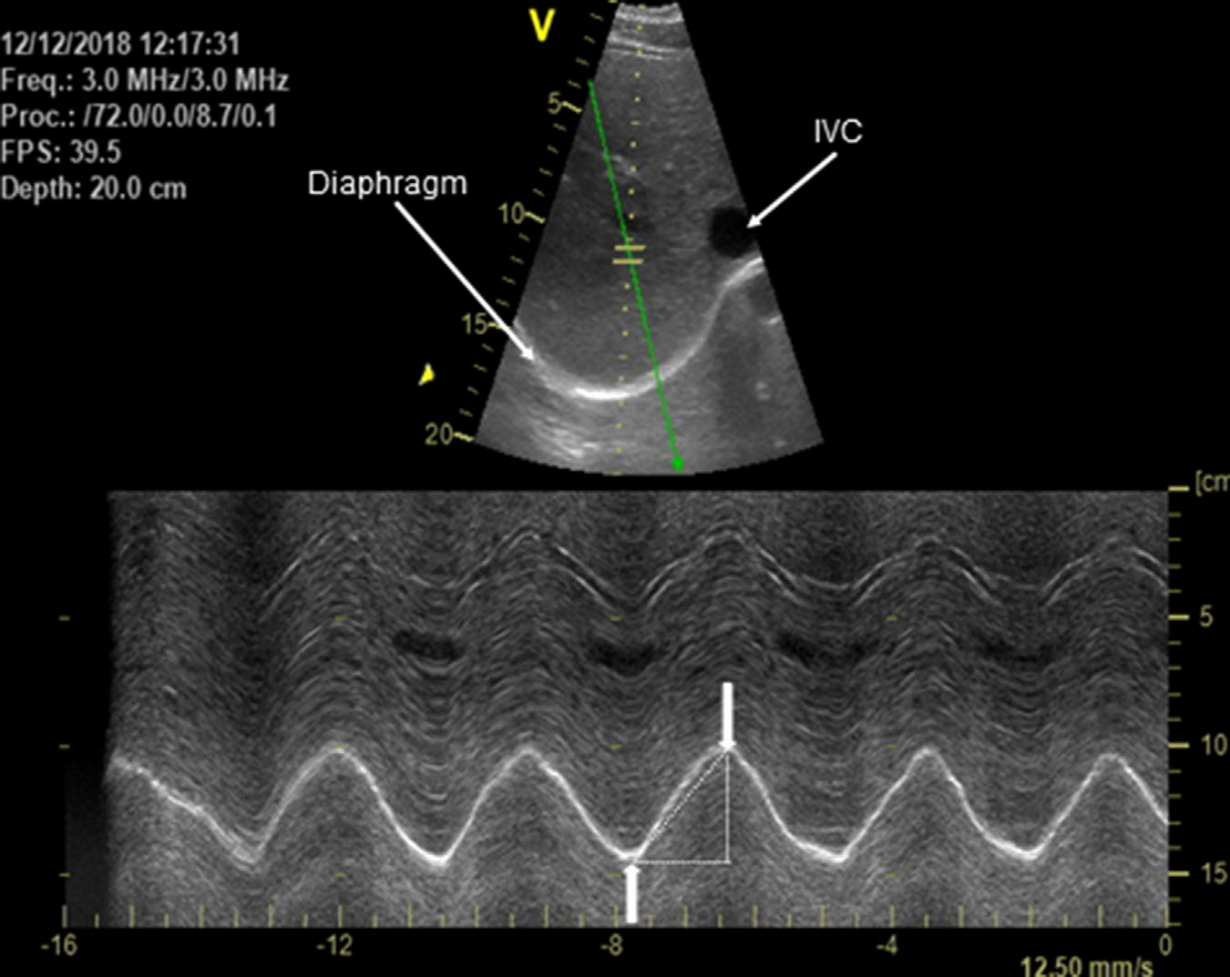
Representative ultrasound images of the right hemidiaphragm during the final 15 s of CO_2_‐rebreathe ($P_{\mathrm{ETC}O_{2}}$, 55 mmHg; ${\dot{V}}_{I}$ 32 l/min). B‐mode image (top) was initially used to obtain the best diaphragmatic delineation, with the inferior vena cava (IVC) used as an anatomical landmark to ensure consistency in the positioning of the transducer. In anatomic M‐mode (bottom), the diaphragm excursion (cm), excursion velocity (slope, cm/s) and excursion time (s) were measured for the estimation of diaphragm work and power output (see text for details). Vertical arrows indicate the beginning and end of a diaphragm contraction
